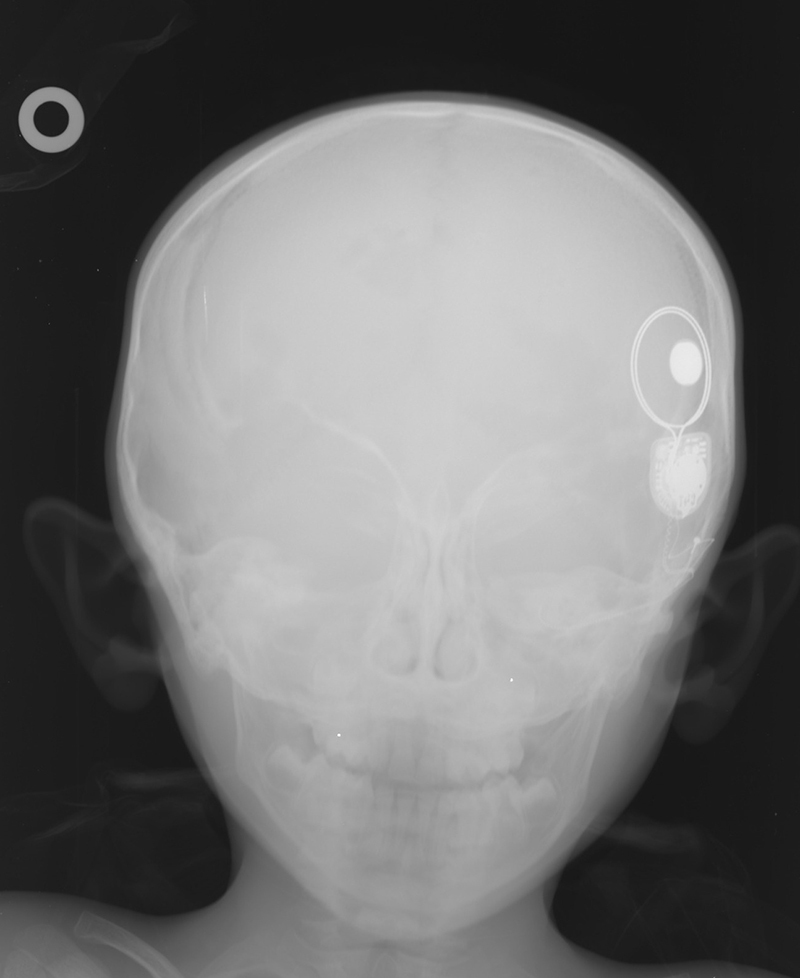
Preoperative radiography of the patient.
